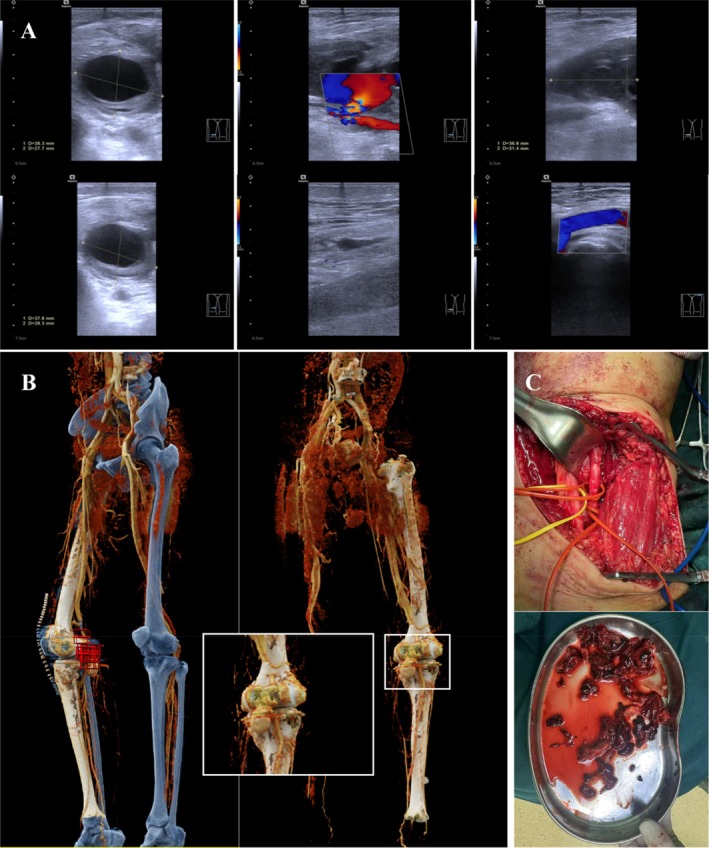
(A) Ultrasound examinations of lower limb vascular structures. A huge mixed‐echo mass was observed at the right popliteal fossa, with a long diameter that could not be measured. The larger transverse plane was approximately 3.78 × 2.83 cm, and the interior contained a dark area. Multiple low echoes were visible at the edges, with a large range of about 3.83 × 2.77 cm. A rupture could be seen in the deep plane connected to the popliteal artery. (B) CTA of the right knee after TKR. Following the bilateral replacement of the right knee joint, metal artifacts interfered with the imaging, making the local display of the popliteal artery unclear. A mass resembling a contrast agent overflow shadow was observed in the popliteal fossa, with a maximum cross‐section of about 32 × 23 mm, locally connected to the popliteal artery. (C): The pseudoaneurysm observed during vascular surgery.
